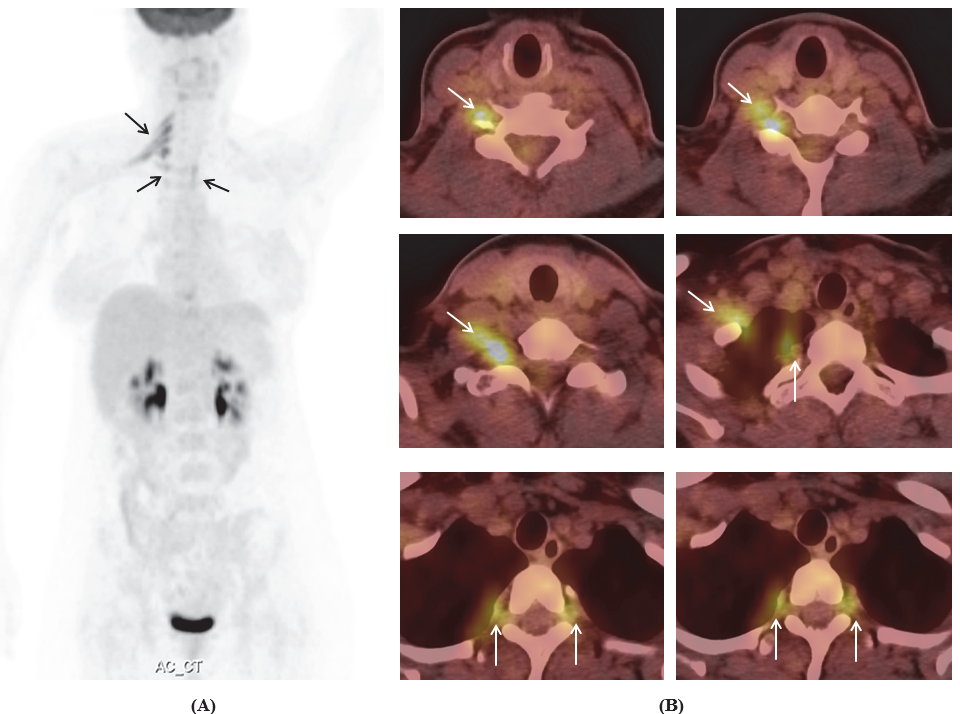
Maximum intensity projection of whole body (A) and PET/CT images (B) showed increased FDG uptake in the right C6, C7, C8, T1nerve roots and branchial plexus, and bilateral T2, T3 nerve roots
